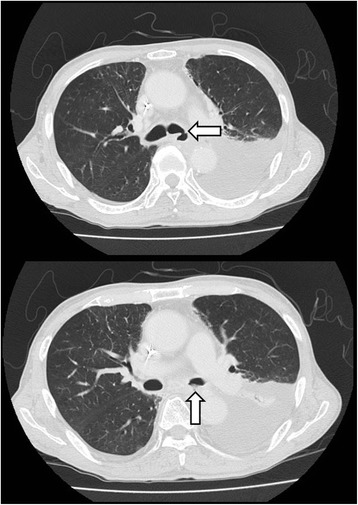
Preoperative computed tomography revealed two esophagobronchial fistulae (*arrows*) at the left main bronchus and pleural effusion in the left thoracic cavity
